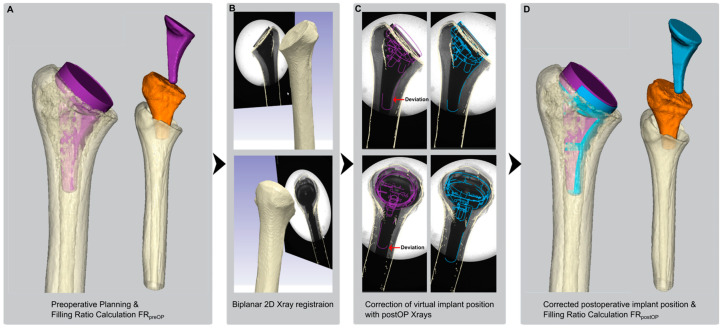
The 2D to 3D registration allowedto validate the accuracy of preoperative canal fill measurements with the actual postoperative implant seating: (A). preoperative planning of the humeral implant (purple) and segmentation of the humeral canal (orange), (B). registration of postOP X-rays, (C). correction of the implant position according to postOP position (blue) and (D). calculation of the true postOP canal fill ratio for comparison with the preOP ratio.
